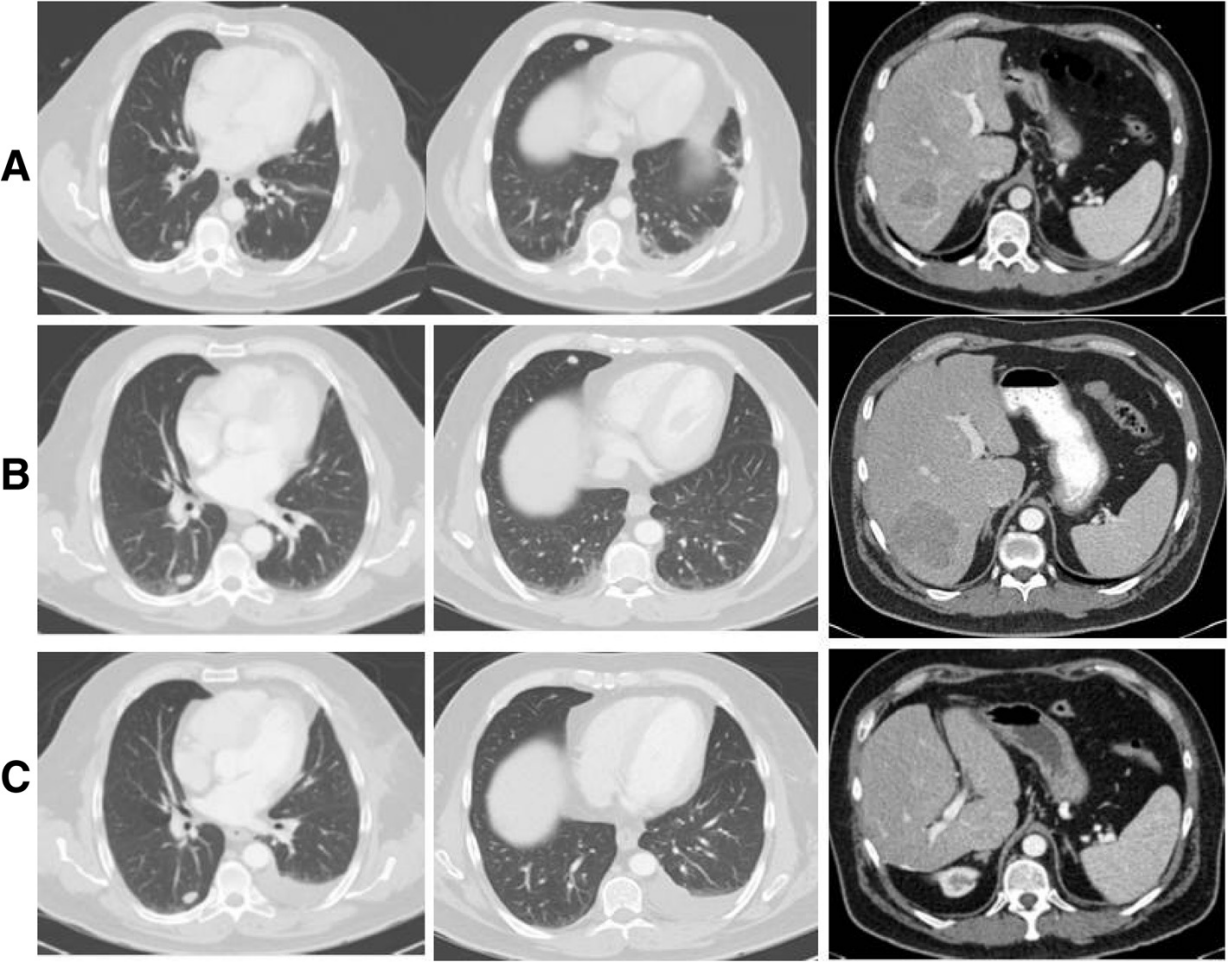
Pre- and post-treatment CT images for patient 1. A. Initial metastatic disease. Small pulmonary nodules on the right anterior and posterior lungs and a 5.4 cm lesion in the right lobe of the liver. B. Post-treatment. Stability or reduction in sizes of right lung lesions, but progression of right lobe of liver lesion to 7 cm. C. Post right hepatectomy. Continued regression and resolution of right lung lesions, and surgical resolution of right liver lesion.
